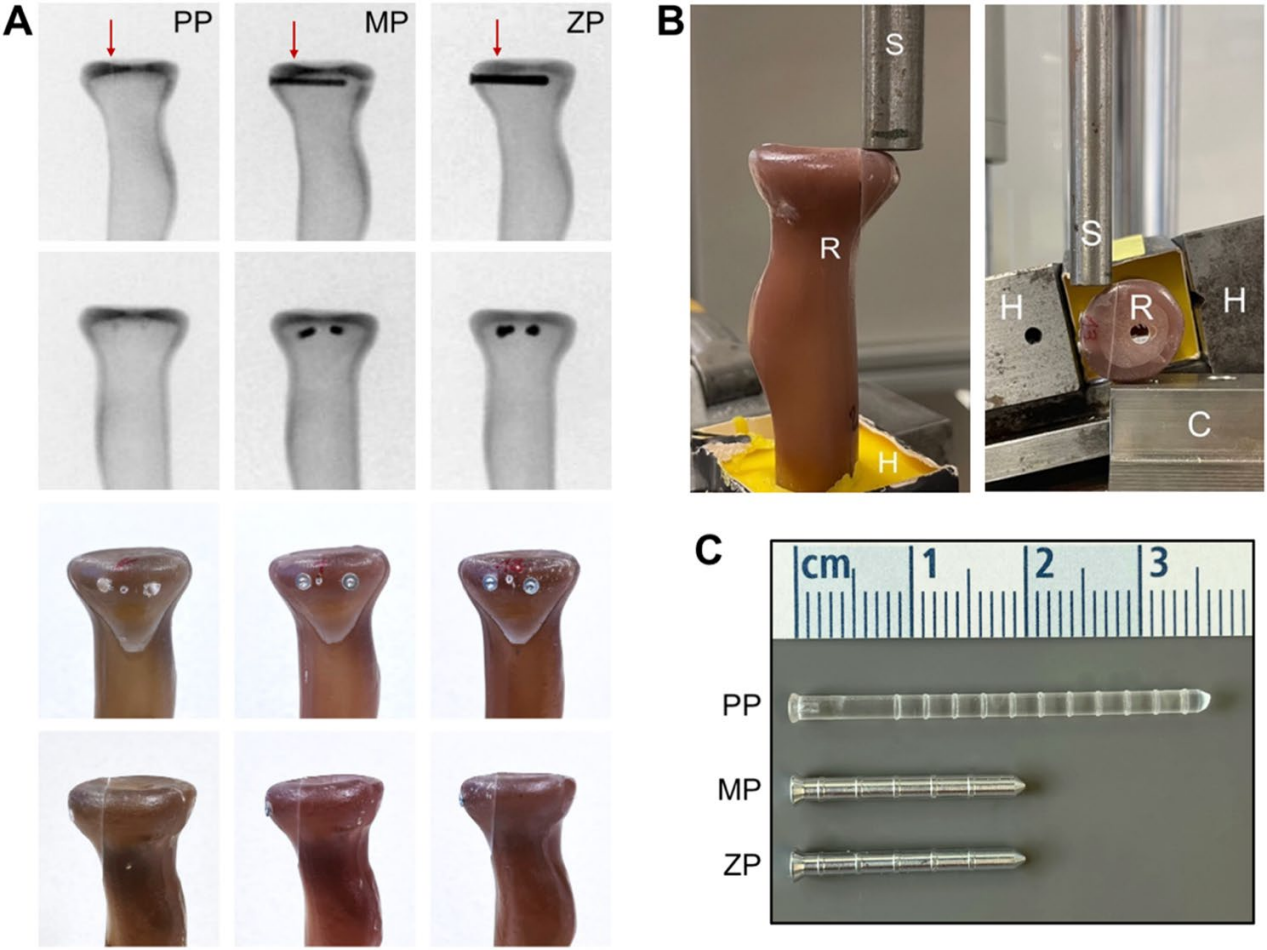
Representative samples, biomechanical loading setup, and osteosynthesis implants. A: Representative samples with red arrows indicating fracture lines. Plain radiography (X-rays) was used for control of fracture replacement and implant placement. B: Biomechanical testing of radius samples (R) mounted to a specific holding unit (H) under axial (left) and transverse (right) loading applied by the hydraulic pressure stamp (S). Note that stamp pressure was applied to the fragment only, parallel to the fracture plane. For transverse loading an additional counterpart (C) was used for sample fixation. C: Representative osteosynthesis implants from the manufacturer. Implants’ length was adjusted to a standardized length of 20 mm for all samples before use. PP, Polylactide Pin; MP, Magnesium Pin; ZP, Zinc Pin
